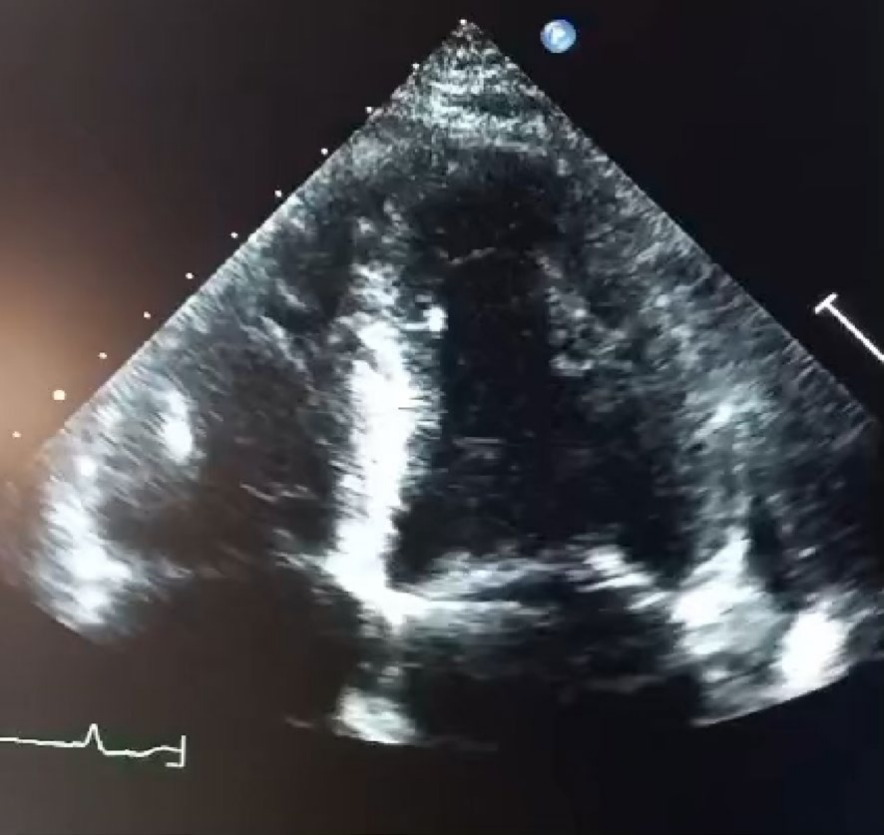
Apical four-chamber view with similar findings
